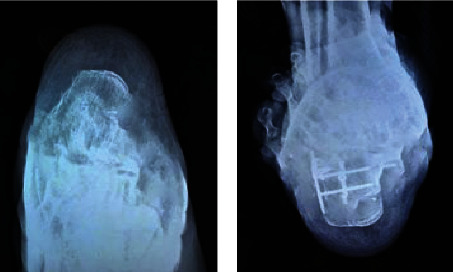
STA surgery: (a) preoperative left calcaneus axial X-ray slice; (b) postoperative left calcaneus axial X-ray slice.
